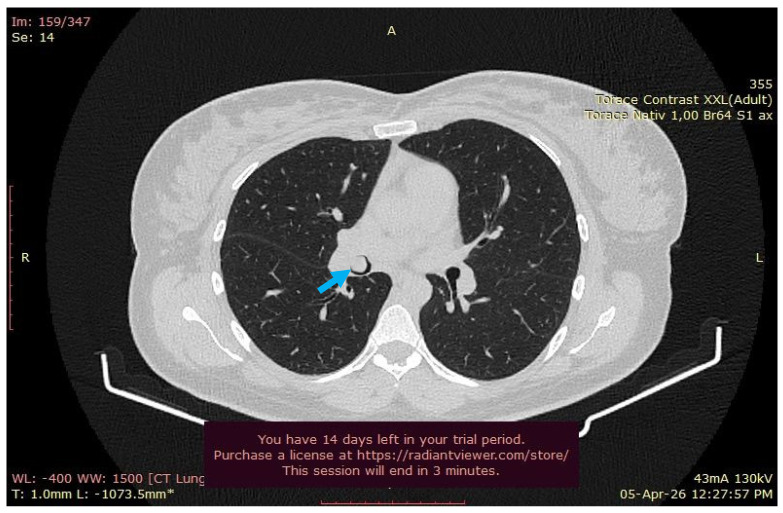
Contrast-enhanced axial chest CT (lung window) demonstrating a large endoluminal endobronchial mass occupying the right main bronchus (blue arrow), with associated bronchial cutoff sign. (Personal archive).
